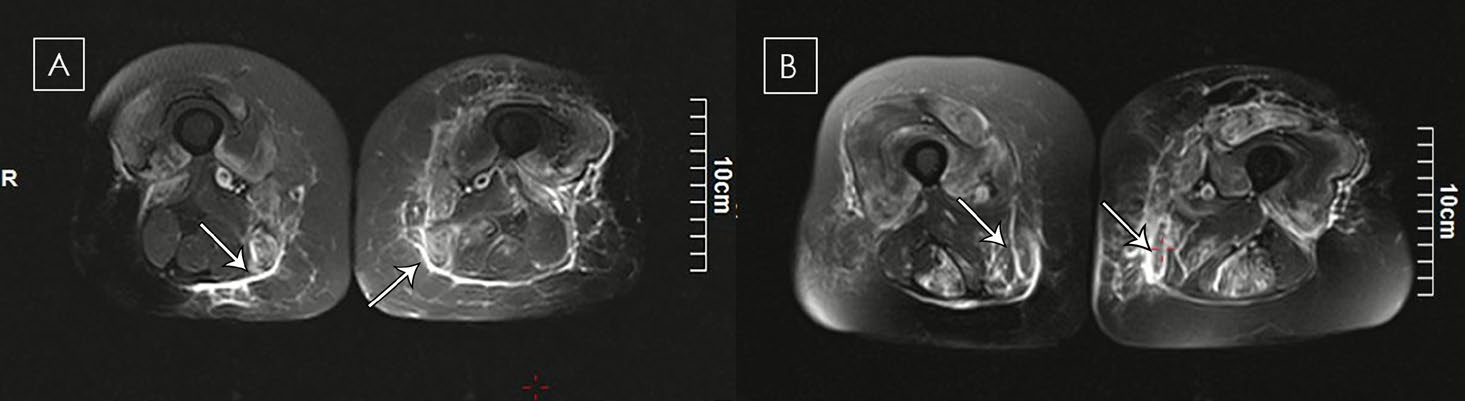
(A) MRI of case 1 revealed mild fatty changes in the sartorius, hamstring, and gracilis muscles. Subcutaneous and soft tissue edema, as well as fascia thickening, are seen in both thighs. (B) There is diffuse, predominant, symmetric, and notable patchy intra‐ and perimuscular edema in both thigh muscles and the lower pelvis. Perimuscular fluid is more prominent along the gracilis and vastus lateralis, as well as along the superficial fascia and minimally along the deep fascia.
